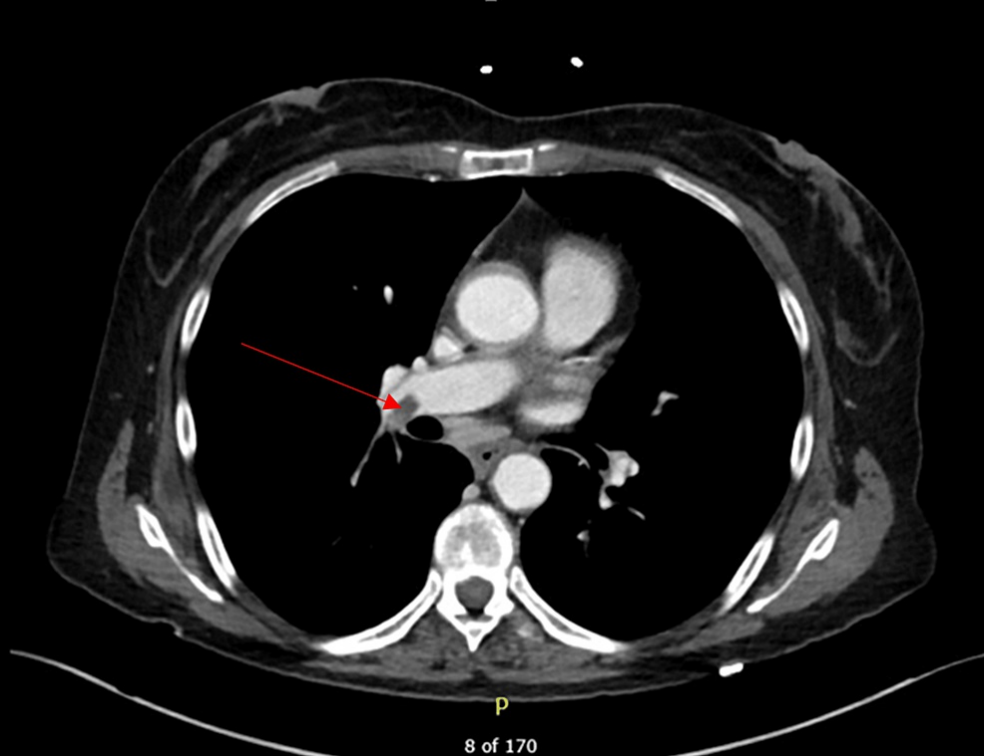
Right-sided pulmonary embolism (indicated by arrow) visualized on CT scan upon patient presentation to the ED
